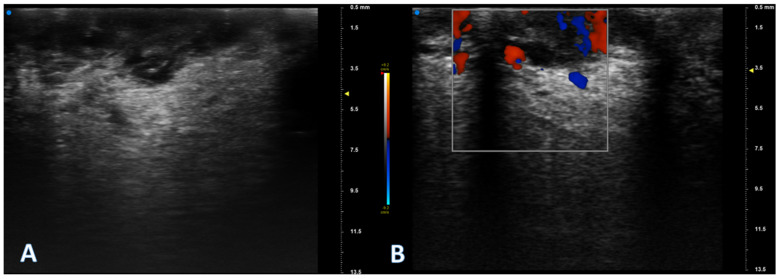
The high-frequency ultrasound (A) reveals an irregular localized thickened area within the oral mucosa presenting as a hypoechoic lesion. The lesion appears to have a slightly irregular surface with the normal free margin presenting as focally interrupted and is confined to the superficial layers of the mucosa. No infiltration into deeper tissues is noted, with globally deeper regular margins. Surrounding tissues show normal echogenicity with no evident signs of metastasis or structural abnormalities. In addition, Doppler signal analysis (B) reveals an increase in vascularity compared to normal tissues. Vascularity is notably peripheral, showing an increase in both arterial and venous components.
